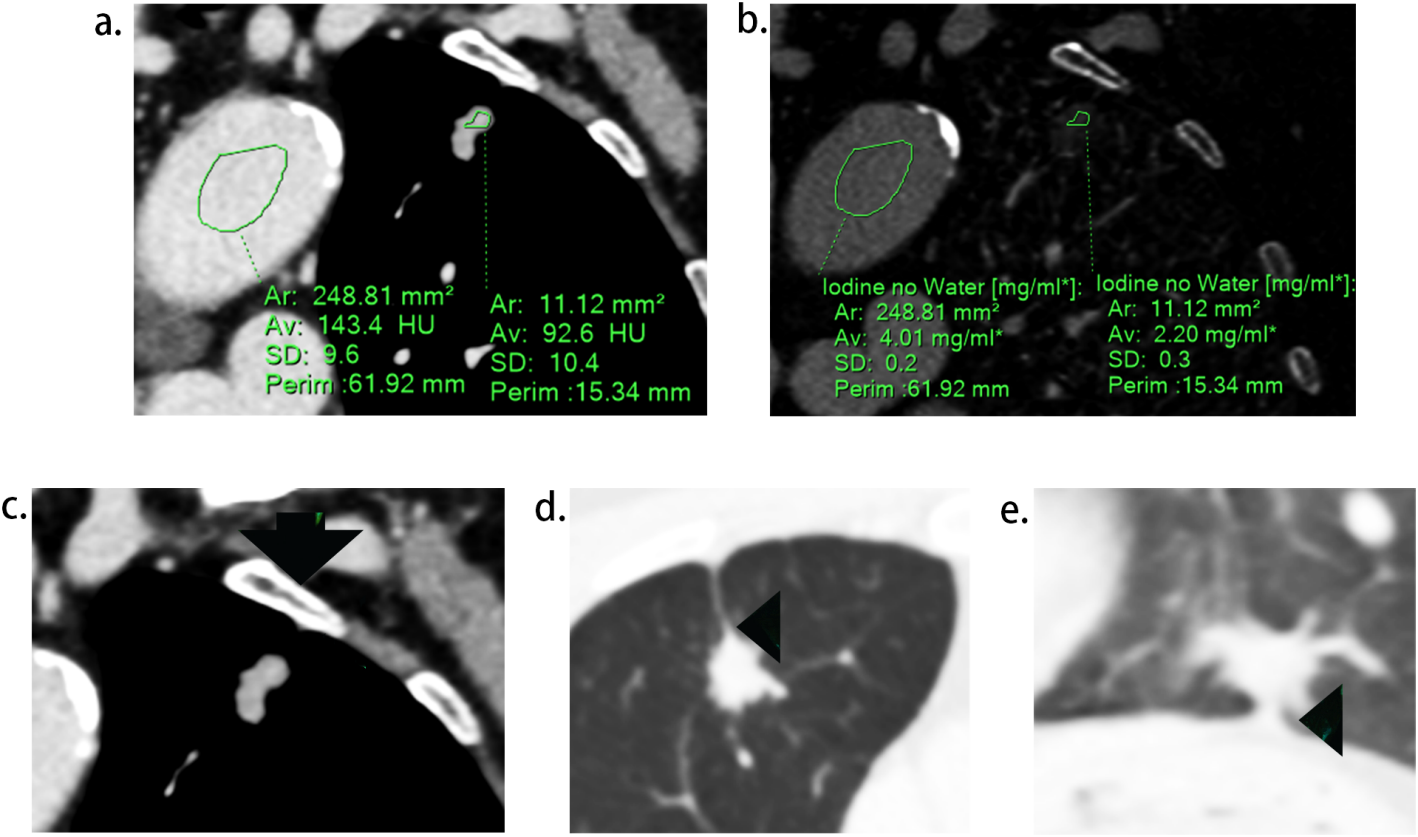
Representative images during CT imaging evaluation. (A), regions of interest (ROI) delineated in lesion and aorta respectively. (B), iodine concentration of the lesion and aorta on the iodine map. (C), soft tissue opacity at the pleural end of the PT (arrow). (D), tumor protrusion (arrow head), defined as triangular opacities extending from the tumor margins to PT. (E), cord-like PT (arrow head), defined as a PT thicker than 2 mm.
